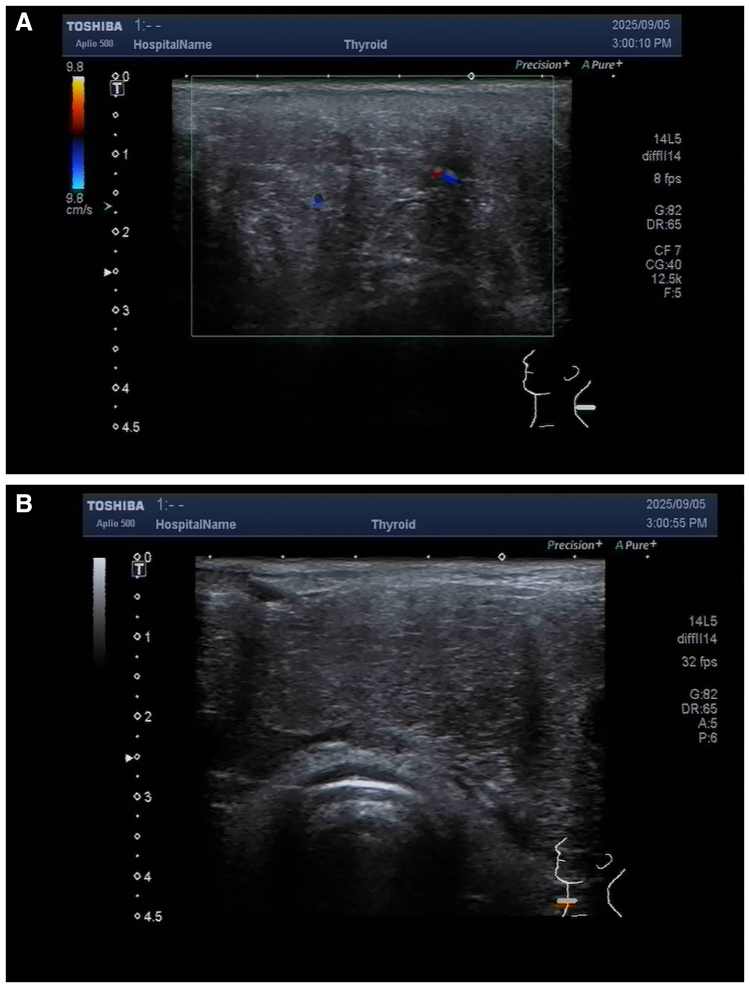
Neck ultrasound images. (A) and (B) demonstrate multiple hyperechoic soft tissue masses in the subcutaneous layer of the neck, without signs of malignant transformation.
